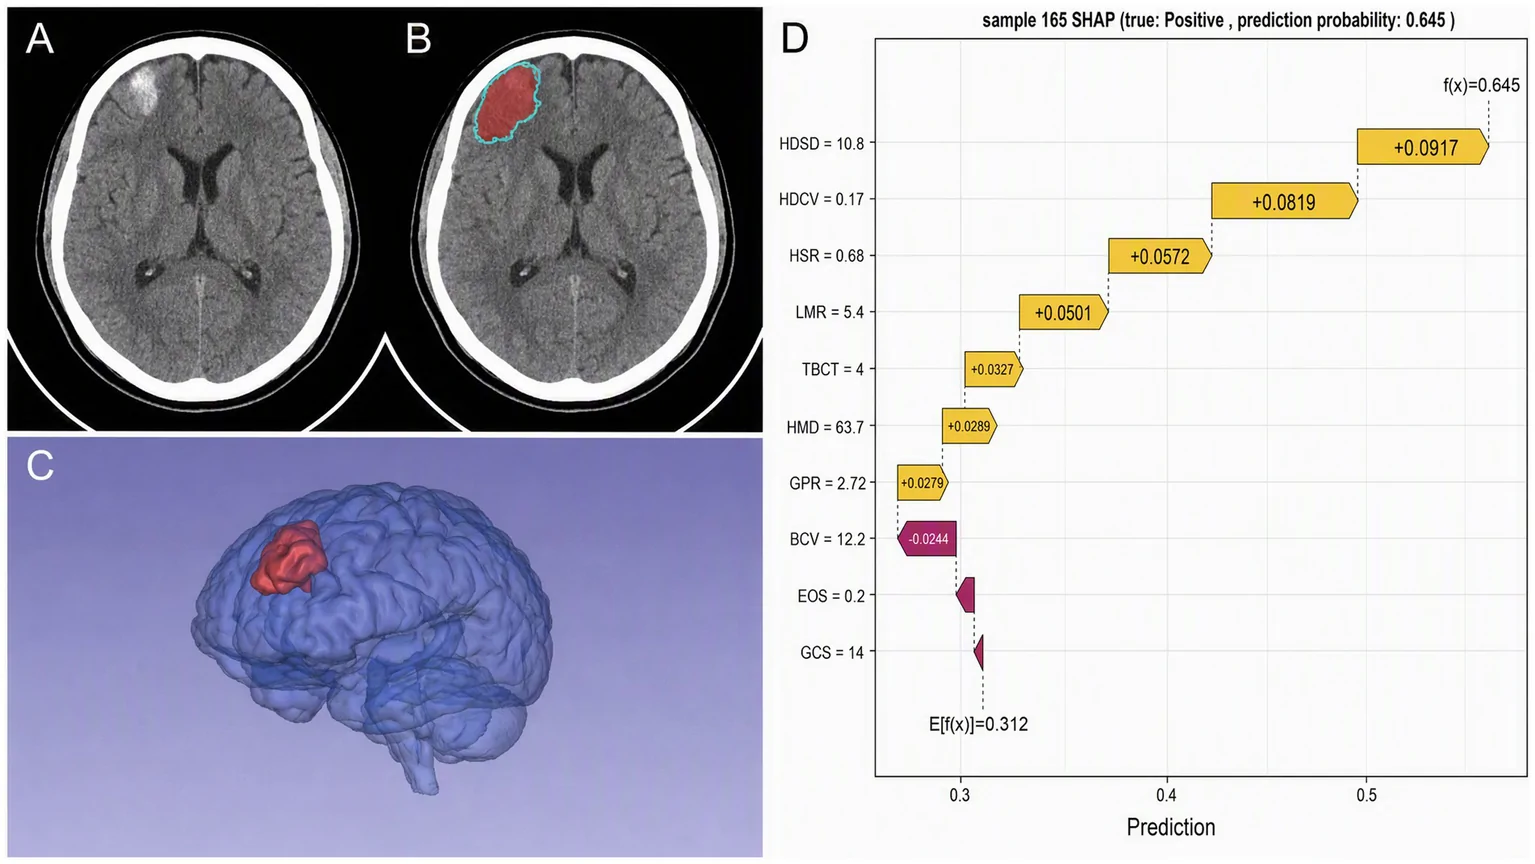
Individual prediction and SHAP interpretation for Patient 165, a patient with frontal lobe contusion and DHP. (A) Baseline CT image. (B) Segmentation of the hematoma and surrounding edema. (C) Three-dimensional reconstruction of the hematoma and surrounding edema. (D) SHAP force plot showing the contributions of individual features to the prediction.
